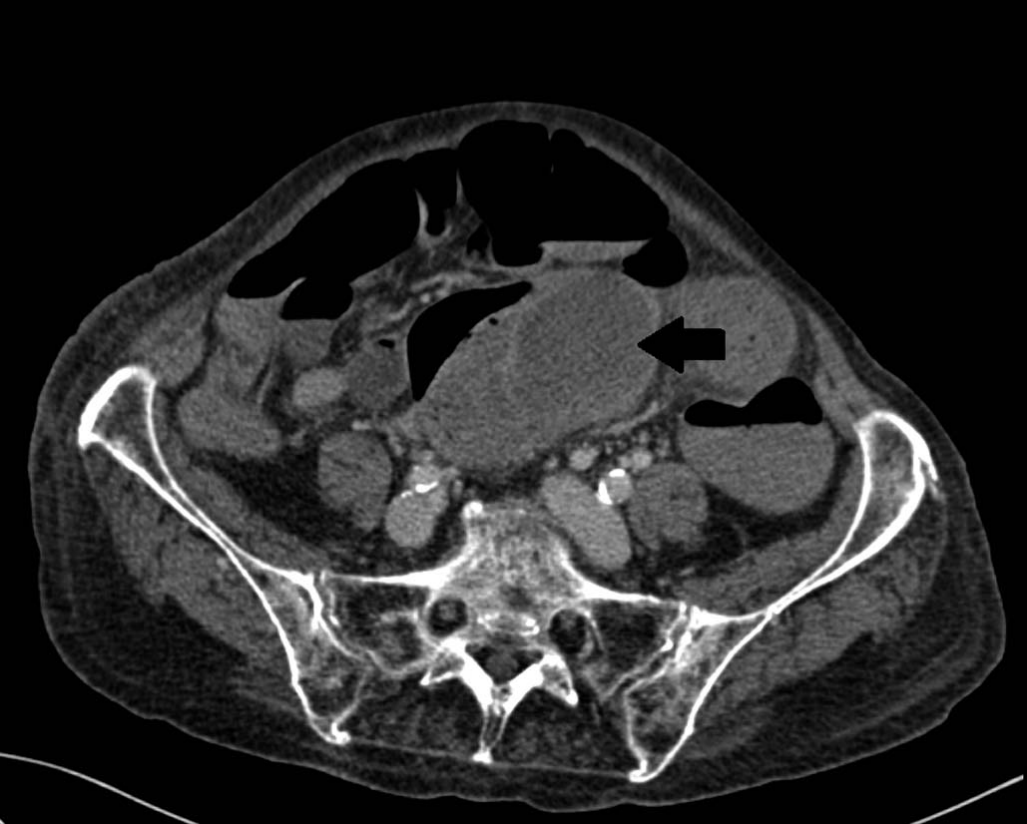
Axial contrast-enhanced computed tomography depicting an intramural hematoma of the sigmoid colon of 70 × 45 × 50 mm and hyperdense material in the lumen consistent with hemorrhagic content.
